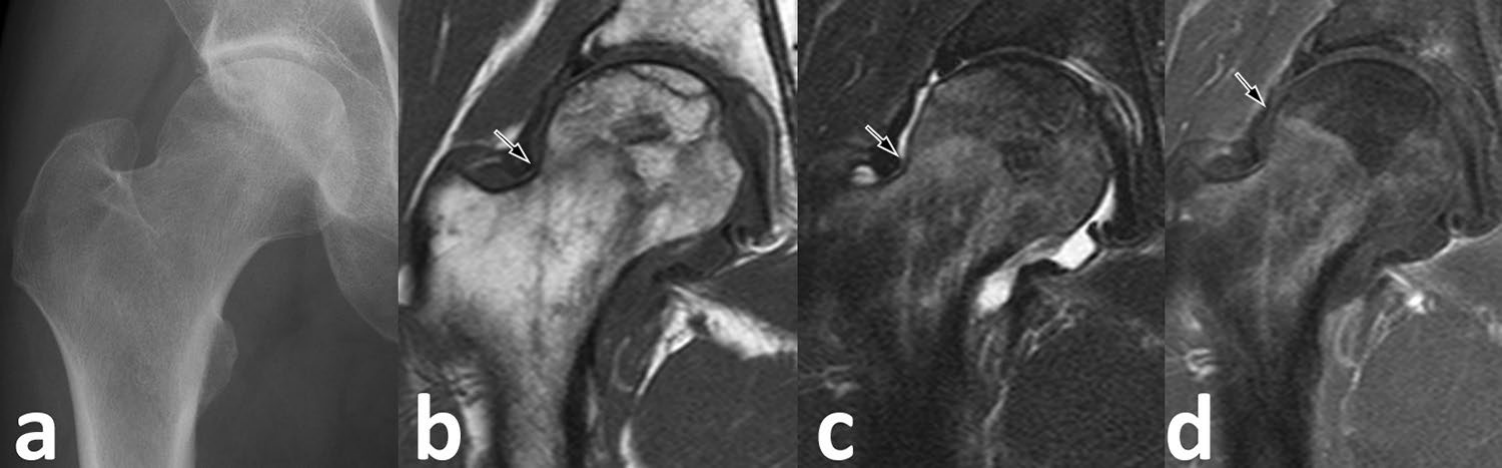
A 43-year-old male with a history of alcohol abuse. (a) An anteroposterior (AP) radiograph of the right hip. An obvious collapse is not observed at the femoral head. (b,c) Diffuse bone marrow edema (BME) secondary to subchondral collapse is observed on unenhanced coronal T1 (b) and FST2 (c) images (ARCO stage IIIA, BME Grade III (b); repetition times/echo times [TR/TE] = 554/10 ms (c); TR/TE = 3300/90). The *arrows* indicate the suspected lateral boundary of the necrotic lesion. The necrotic angle is 142° on the T1 and 139° on the FST2 images. (d) The contrast-enhanced MR image (TR/TE = 628/10) shows a more medial boundary (*arrow*), and the necrotic angle is 120°.
